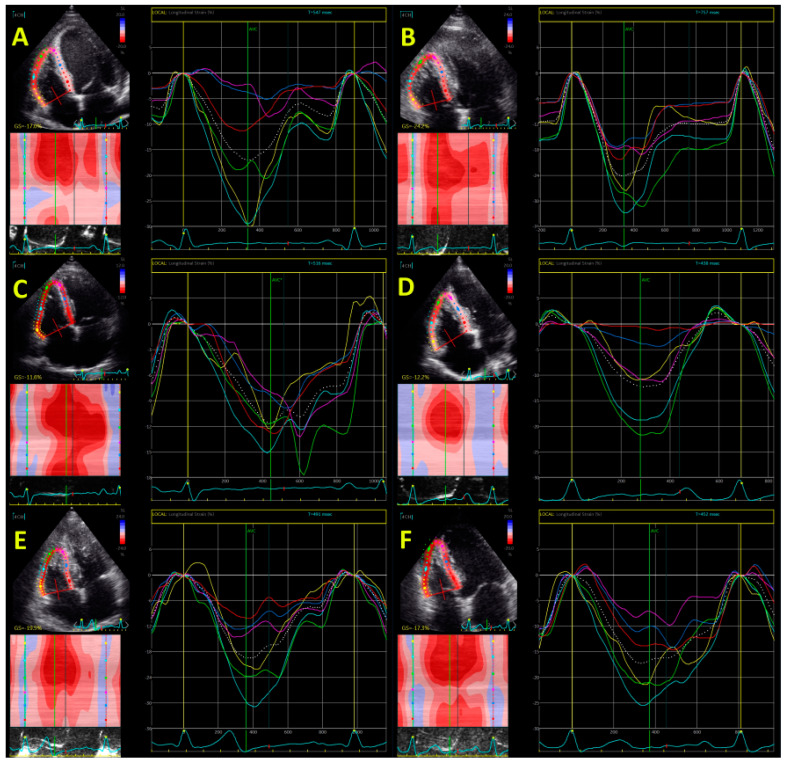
Echocardiography-derived right ventricular global longitudinal strain in patient with dilated cardiomyopathy (panel A); hypertrophic cardiomyopathy (panel B); arrhythmogenic right ventricular cardiomyopathy (panel C and D); amyloidosis (panel E); and acute myocardial infarction (panel F).
